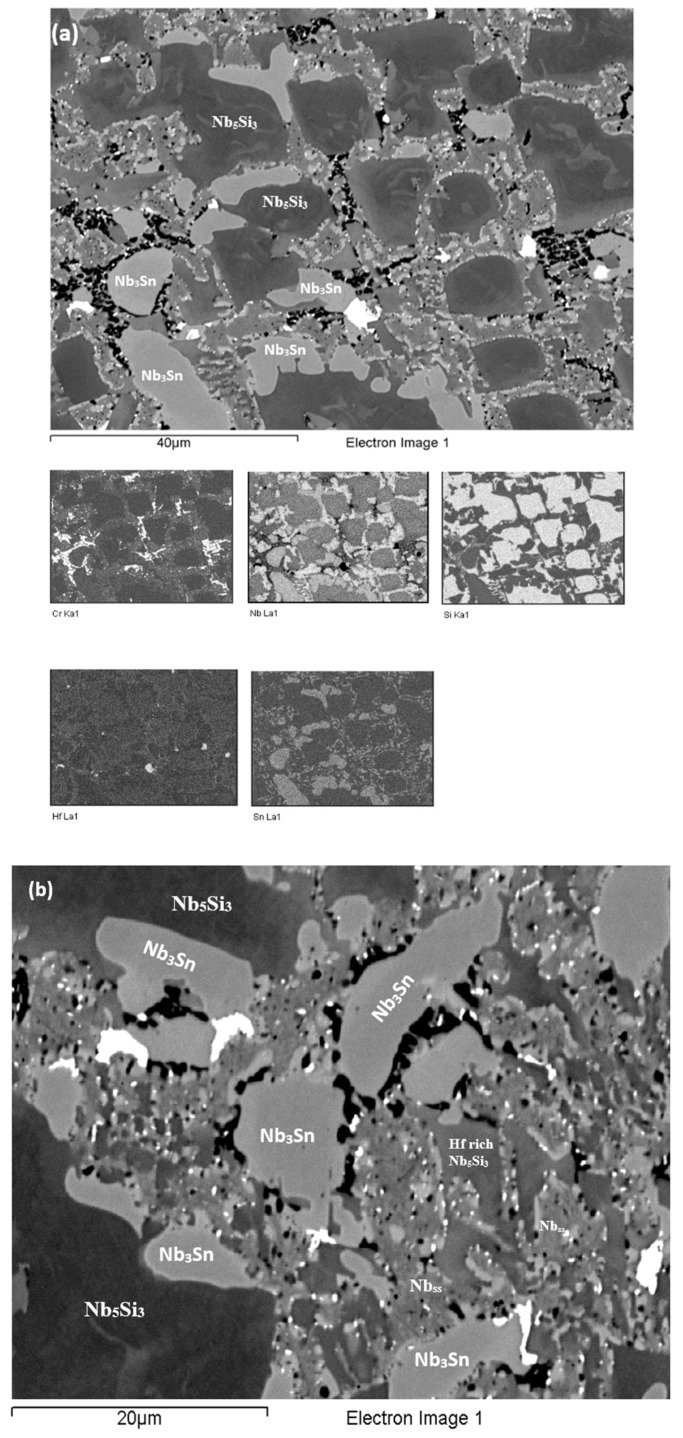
BSE images of the EZ3-HT (1200 °C/100 h) of (a) of the bulk with X-ray elemental maps and (b) details of an area with the C14-NbCr_2_ Laves phase.
